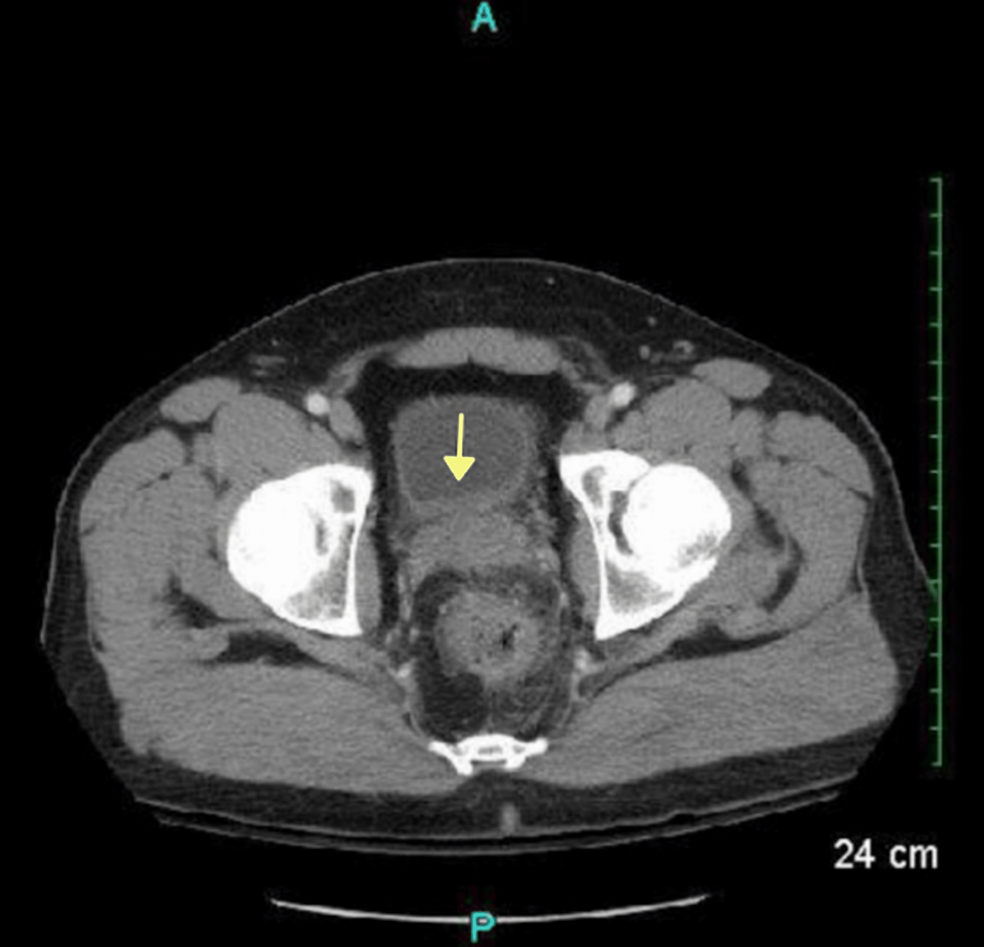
Computed tomography (CT) with intravenous (IV) contrast scan demonstrating pockets of air and irregular hyper-dense nodular material in the anterior bladder and diffuse urinary bladder wall thickening (yellow arrow)
